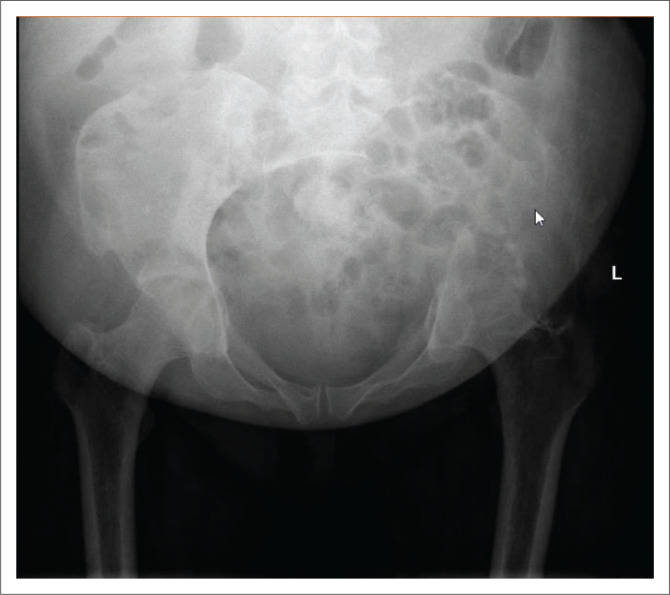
An X-ray demonstrated an absent left hemipelvis.
